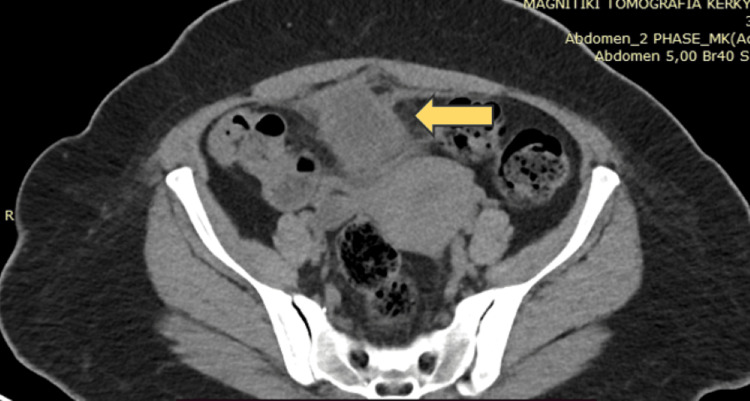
CT scan revealed a lesion suggestive of possibly cystic tissue
